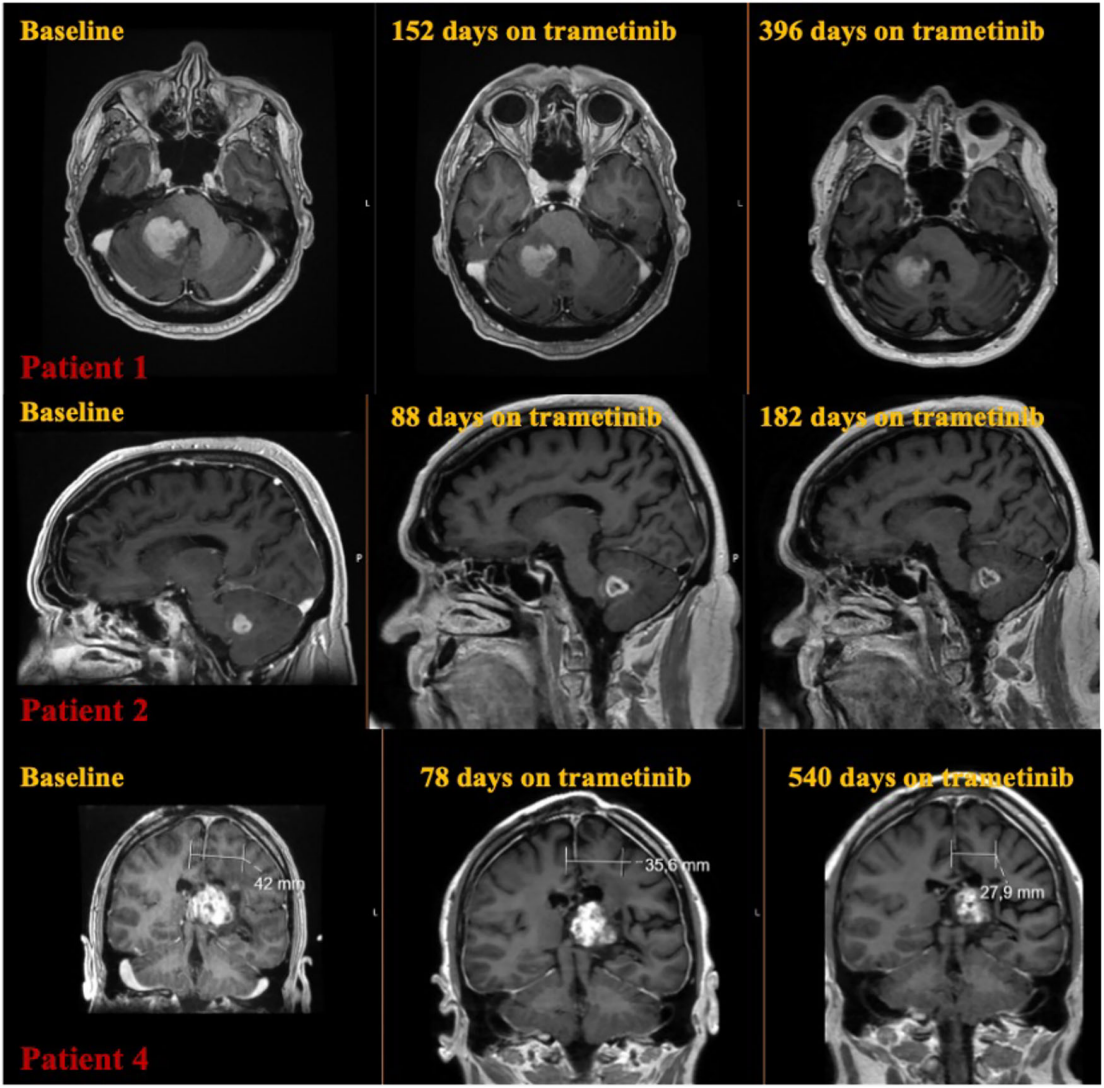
T1 weighted MRI images with contrast enhancement demonstrating the fluctuation of tumor growth in patients 1, 2, and 4 of our cohort under trametinib treatment. Baseline MRI means MRI control before the start of MEK inhibitor.
